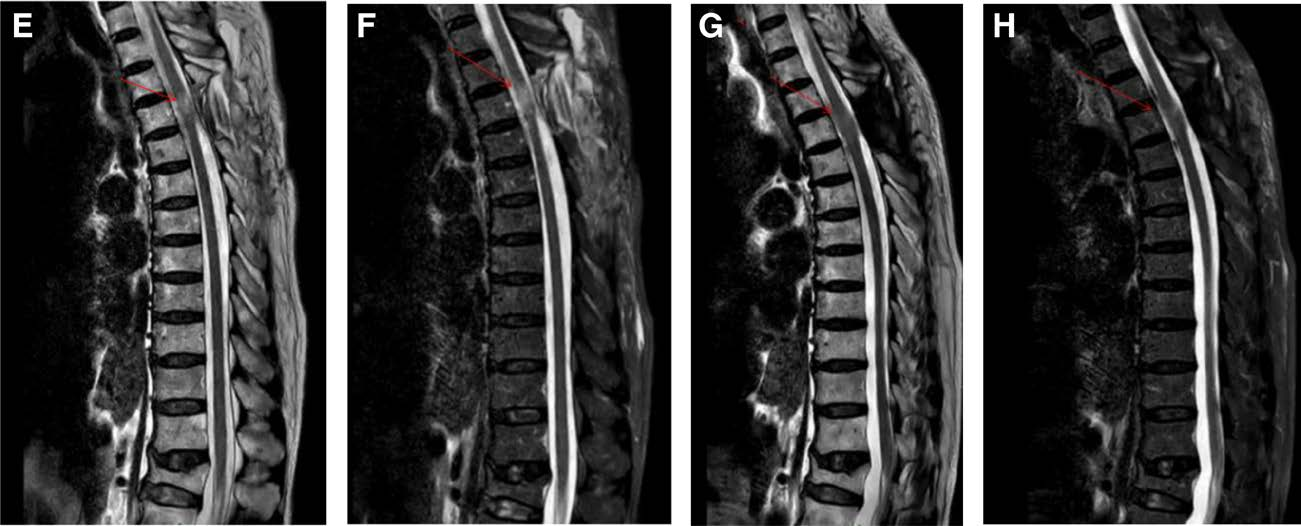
Postoperative imaging date: (E and F) Postoperative changes in sagittal MRI of the thoracic 3 to 5 vertebral canal after removal of the drainage tube, relief of spinal cord compression at the level of the thoracic 4 vertebral body, and spinal cord degeneration; (G and H) sagittal MRI at 2 months after surgery: Postoperative changes in the posterior spinal canal of the thoracic 3 to 5 vertebrae, reduced swelling of surrounding soft tissue compared to before, relieved compression of the spinal cord at the level of the thoracic 4 vertebrae, and spinal cord degeneration. MRI = magnetic resonance imaging.
